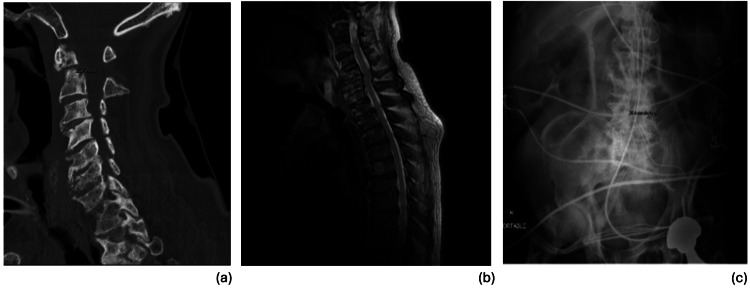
Significant radiological findings during hospital course (a) CT cervical spine demonstrates Type II fracture of the C2 vertebra with approximately 2.7 mm of superior distraction of the dens fracture fragment; (b) MRI cervical spine demonstrates fluid cleft at the known non-united type II odontoid fracture. There is abnormal cord signal at the cervicomedullary junction, with small rim-enhancing area, representing evolving hemorrhagic contusions; (c) Abdomen X-Ray demonstrates questionable short segment of large bowel with featureless wall in the mid abdomen, which can be seen in the setting of ischemia.
